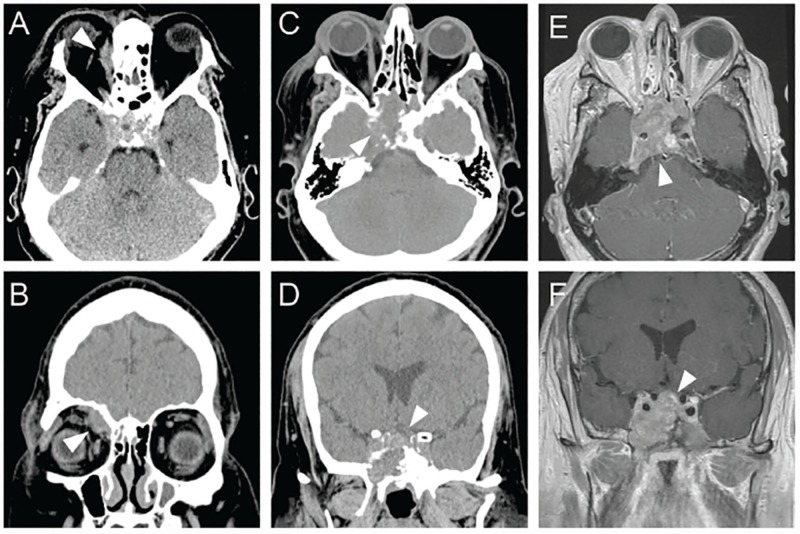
Patient progression from Cushing disease Non-contrasted axial (A) and coronal (B) tissue-windowed head CT images highlighting right superomedial right orbital abscess (arrowheads). Non-contrasted axial (C) and coronal (D) tissue windowed head CT images and gadolinium-contrasted T1-weighted axial (E) and coronal (F) brain MR imaging revealing invasive pituitary macroadenoma with suprasellar extension and local bony destruction (arrowheads).
